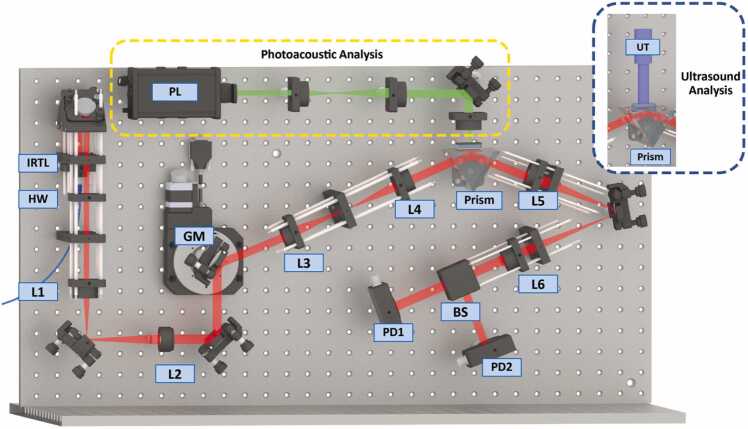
Experimental setup. IRTL: infrared tunable laser; HW: half waveplate; GM: galvomirror; PL: nanosecond pulse laser; UT: ultrasound transducer; BS: beam splitter; PD: photodetector.
